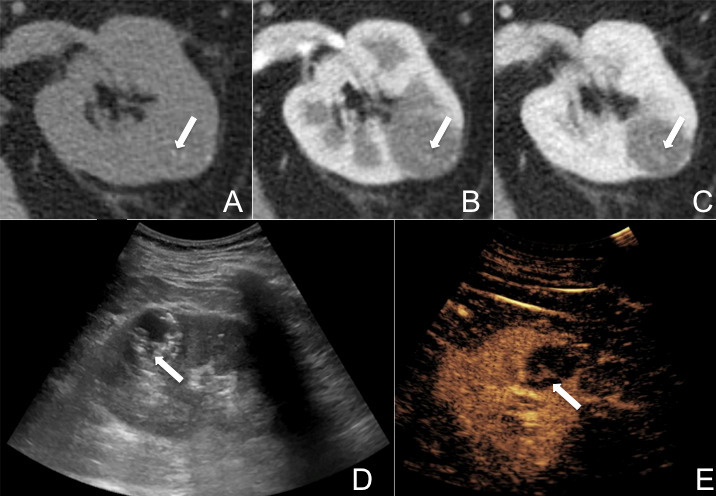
Reclassification of a CRC from Bosniak 2F (initial CT categorization) to Bosniak 3 category (final CEUS categorization). CT found a well-defined round mass in the left kidney, showing fluid attenuation and thin incompletely calcified septa on both unenhanced scan (arrow in A) and post-contrast arterial and venous phases (arrows in B and C). On preliminary B-mode ultrasound, a complex cysts with hyperechoic components was found (arrow in D), some of which showed measurable enhancement during CEUS evaluation (arrow in E). The lesion was referred to surgical treatment with pathological diagnosis of multilocular cystic renal neoplasm of low malignant potential. CEUS, contrast-enhnaced ultrasound; CRC, complex renal cyst.
